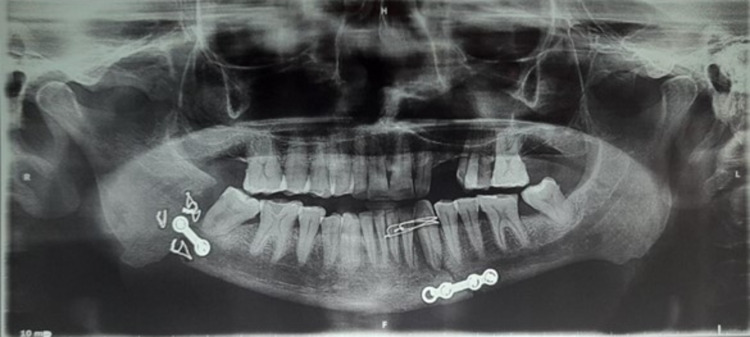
Pre-operative orthopantomogram (OPG) showing the discontinuity of the bony fragments at the right angle region of the mandible
